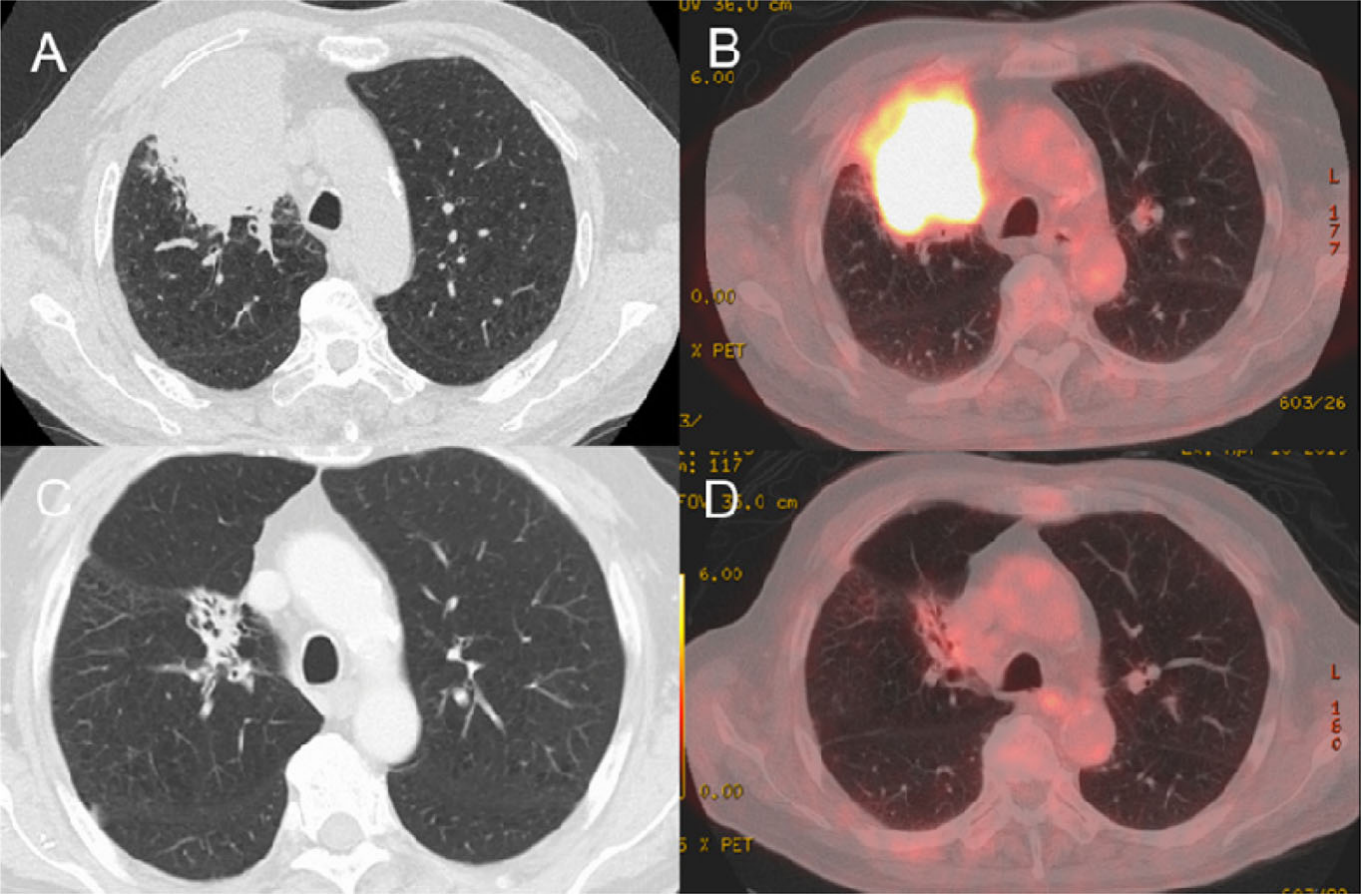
A mass with a size of 85 mm is shown on chest computed tomography (CT) (A). Intense uptake is shown in the lesion visualized with 18F‐fluorodeoxyglucose positron emission tomography (PET) (B). Chest CT and PET‐CT show a significant reduction in the size of the mass compared with that observed 15 months earlier (C,D).
